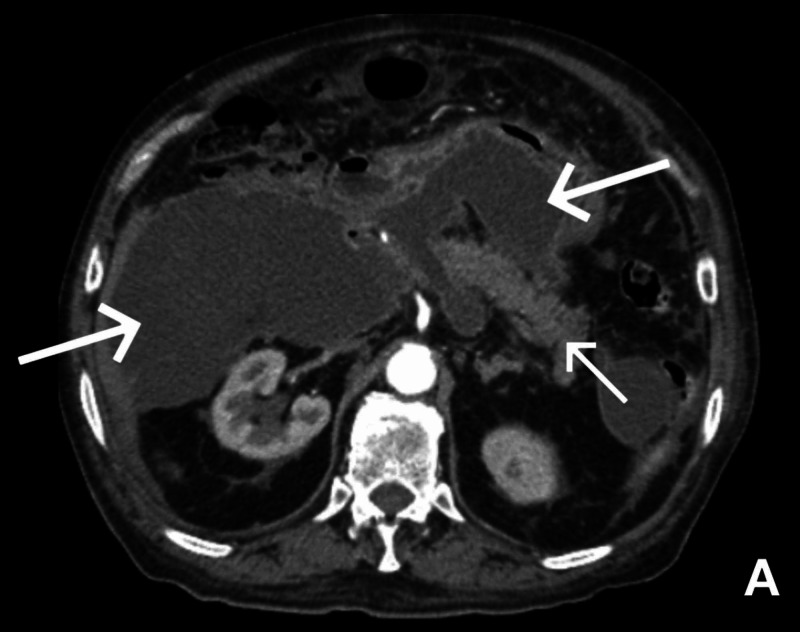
CT abdomen/pelvis with IV contrast and arrows showing multiple loculated fluid collections concerning for pancreatic pseudocysts vs abscesses. Smaller arrow is pointing to the pancreas while larger arrows are pointing to the fluid collections.
